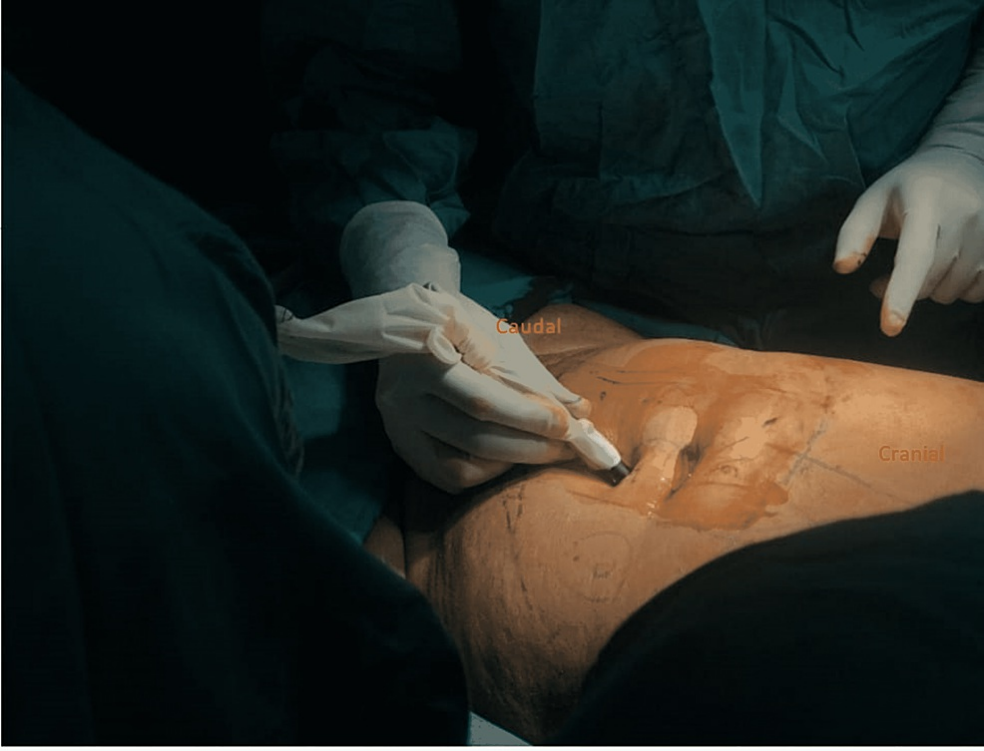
Identification of inferior epigastric artery with Doppler
